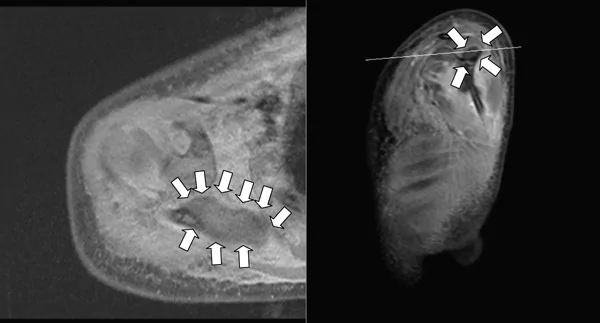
T1 fat saturation postcontrast MRI of the right shoulder with and without contrast demonstrating an abscess within the supraspinatus fossa measuring 3.6 by 1.5 by 0.9 cm causing significant myositis in the supraspinatus muscle belly. Abscesses outlined in white arrows.
